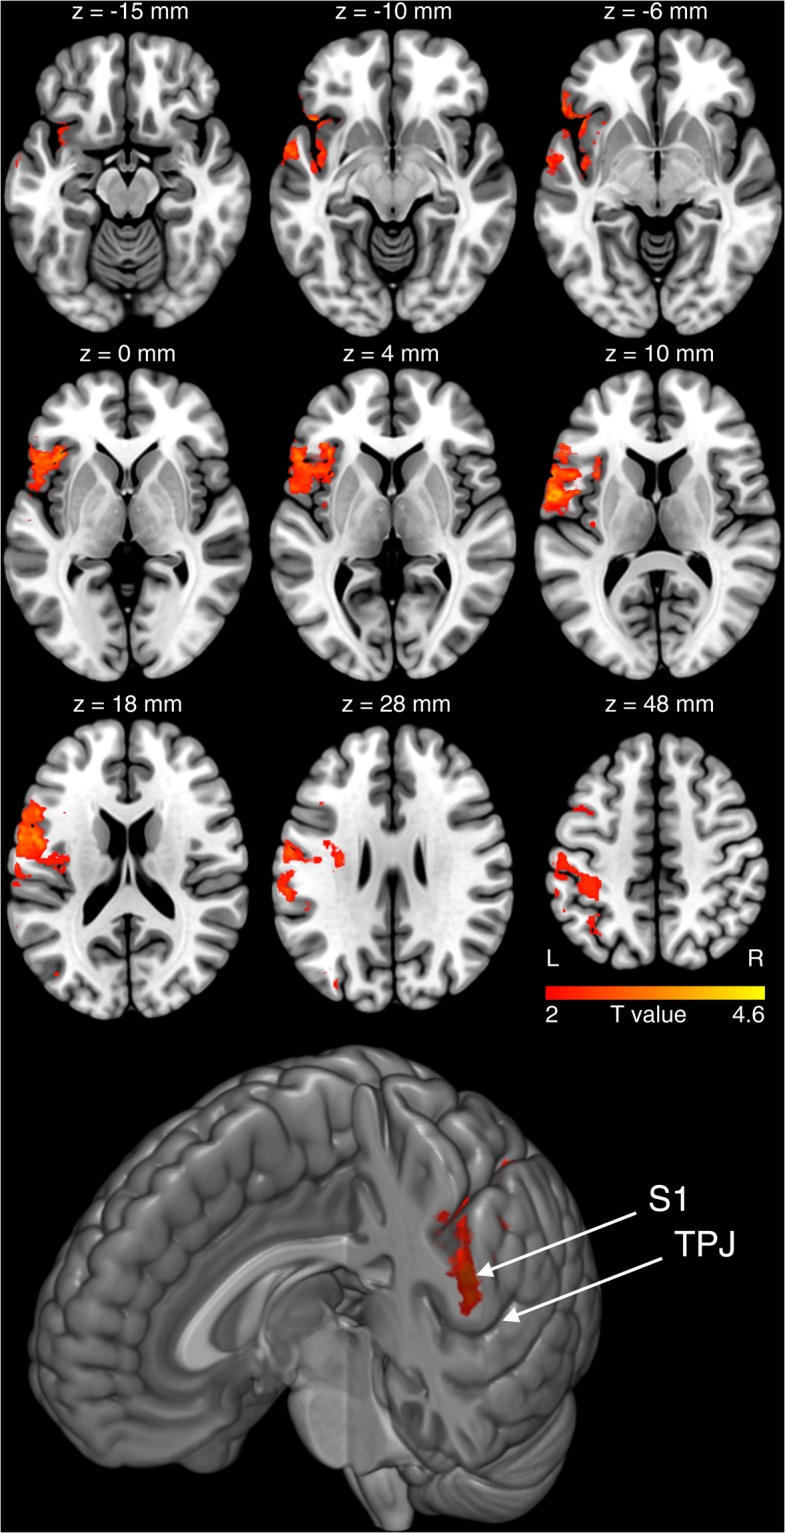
MRI-based lesion symptom mapping. Shown are the brain regions that overlap with an impaired fat perception in the category f3. For f1, we also found significant differences in fat ratings between patients and controls. VSLM, however, revealed no significant effects for f1 (see Fig. 1 for corresponding sugar and fat ratings). The ‘z’ below each brain slice indicates the corresponding z-coordinate of that slice in MNI space. ‘L’ indicates the left and ‘R’ the right hemisphere. We found that an impaired fat perception in f3 overlapped with the anterior insular cortex together with the frontal operculum. Both regions are together assumed to underpin gustatory processing. We also found the mid-dorsal insula together with the primary and secondary somatosensory cortex - regions that are assumed to integrate sensory-oral inputs. At the bottom of the figure we show a render brain with a coronal cut through the primary somatosensory cortex. TPJ indicates the temporoparietal junction, S1 the primary somatosensory cortex. The effect in f3 appears to overlap with the representations of the face including the oral cavity as well as the tongue.
